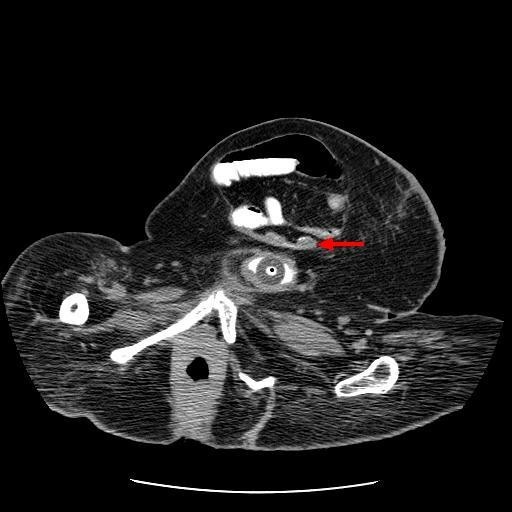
Axial computed tomography of abdomen, performed on 19 June 2007, revealed midline, lower abdominal wall hernia, whose neck measured 45 millimetres wide. Within the hernia, were several loops of small bowel and vesicostomy. (arrow).
